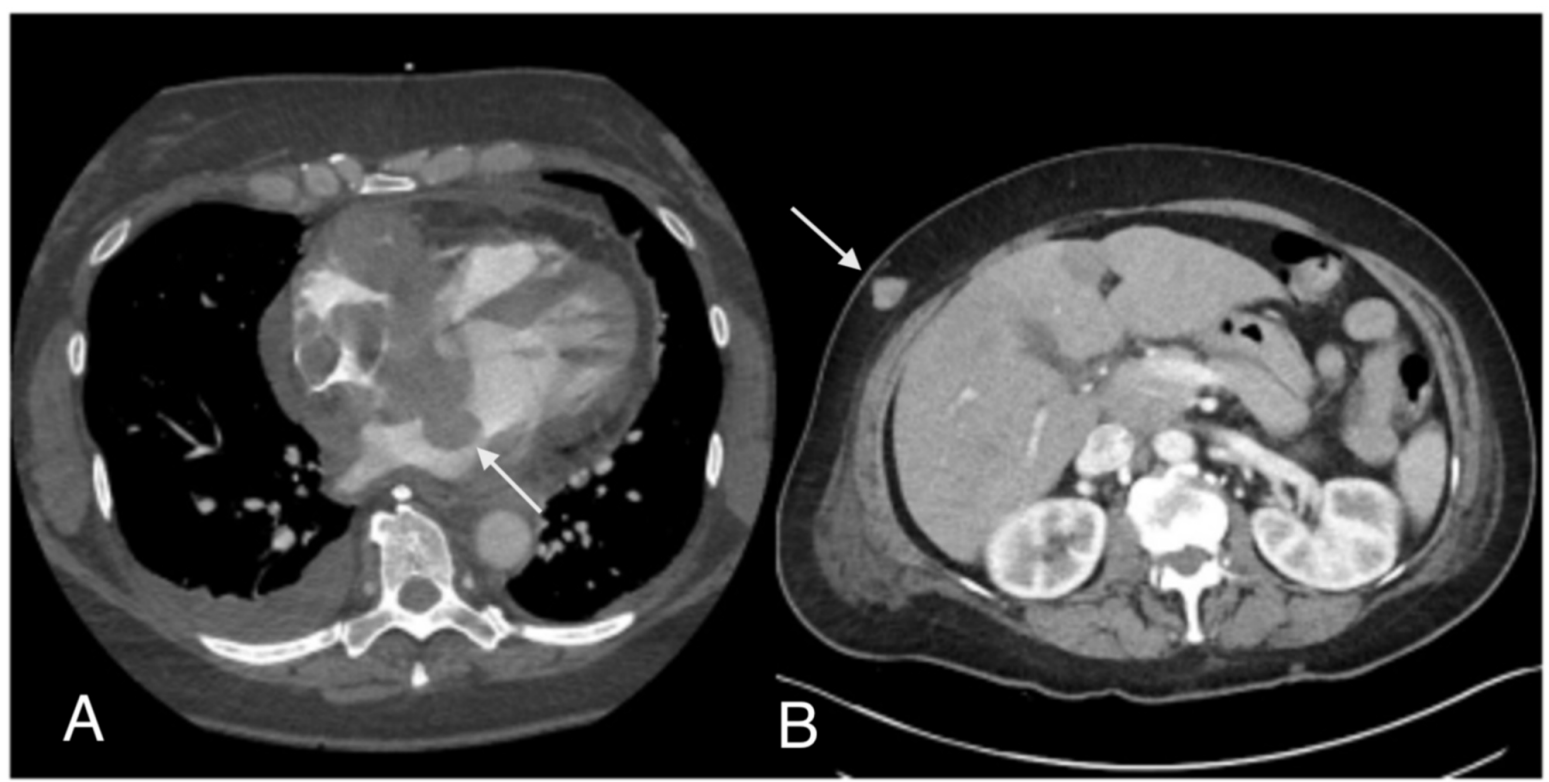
CT abdomen and pelvis with IV contrast. Left panel: Interatrial septal cardiac masses; Right panel: Abdominal wall mass
